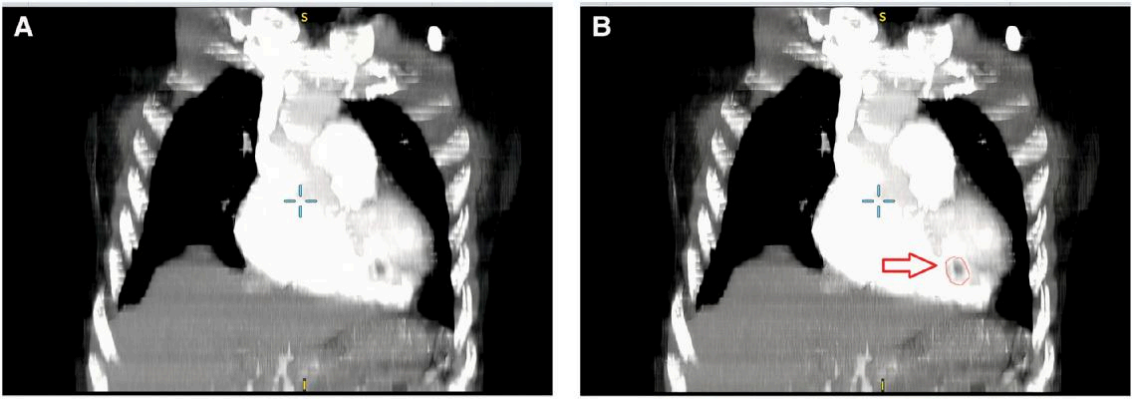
Contrast-enhanced chest CT showing severe right heart strain (RV/LV ratio >1) and dilated pulmonary artery (37 mm).
